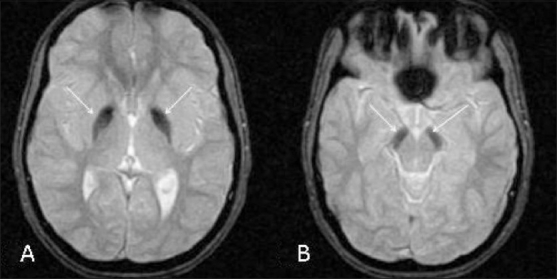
Axial T2W gradient-recalled echo MRI images show marked hypointensity in the globus pallidi (arrows in A) and parsreticulata of the substantia nigra (arrows in B)
